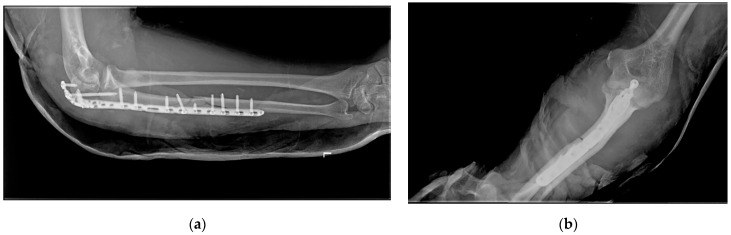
Follow-up radiograph at two weeks: (a) lateral view with forearm in neutral position; (b) anterio-posterior view with forearm in neutral position.
